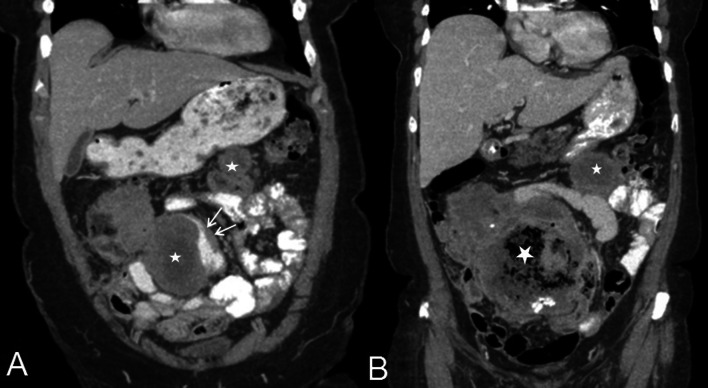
a, b 78-year-old male with biphasic peritoneal mesothelioma. Serial CT scans show rapidly progressive disease. a Coronal CT showing large soft tissue mass in the mesentery (*) indenting and distorting the small bowel and causing eccentric bowel wall thickening (arrows). b Coronal CT section through the same site three months later shows increase in the size of the mass and air pockets within suggestive of contained bowel fistulation and disease progression
